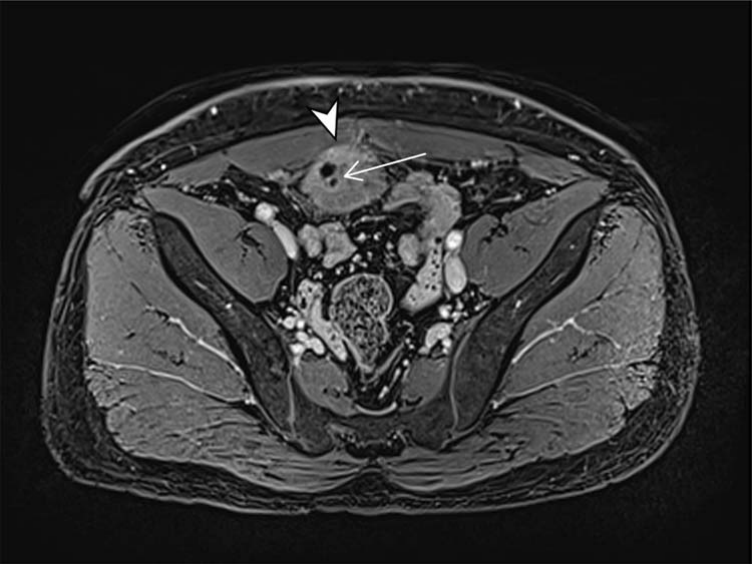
Axial MRI image of lesion. Arrowhead indicated involvement with the rectus abdominis. Arrow indicates lesion and calcific focus.
